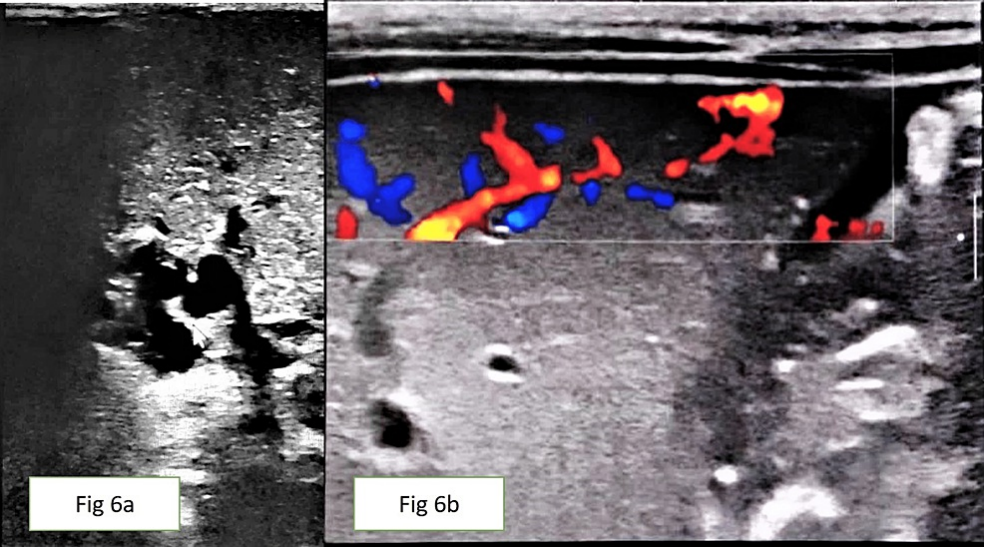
A known case of Caroli's disease: a) Ultrasound image demonstrating multiple intrahepatic cystic dilatations of the biliary tree; b) Doppler image demonstrating the presence of hepatic subcapsular flow
